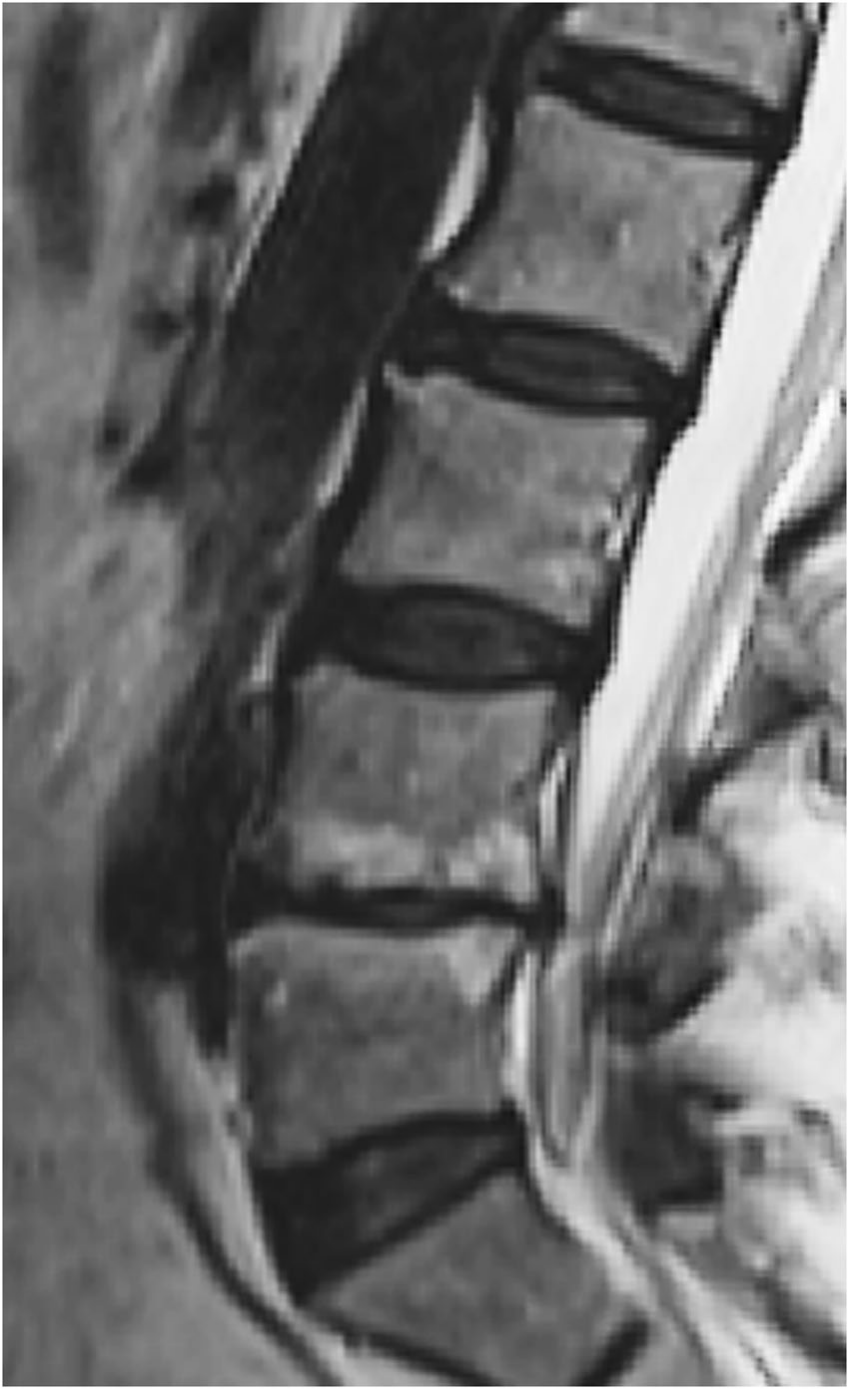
MRI scan showing endplate defect grade VI both at the L4/L5 rostral and caudal endplates, with associated Modic changes (MC) over both rostral and caudal bone marrows adjacent to endplates and disc degeneration evaluated as Pfirrmann grade 5. As the endplate is a fundamental part of the vertebral body-endplate-intervertebral disc motion segment, one could consider endplate defects to be an initiating factor not only for disc degeneration, but also for MC. MRI indicates magnetic resonance imaging. From Rade M, *et al*. Vertebral endplate defect as initiating factor in intervertebral disc degeneration: Strong association between endplate defect and disc degeneration in the general population. *Spine*
